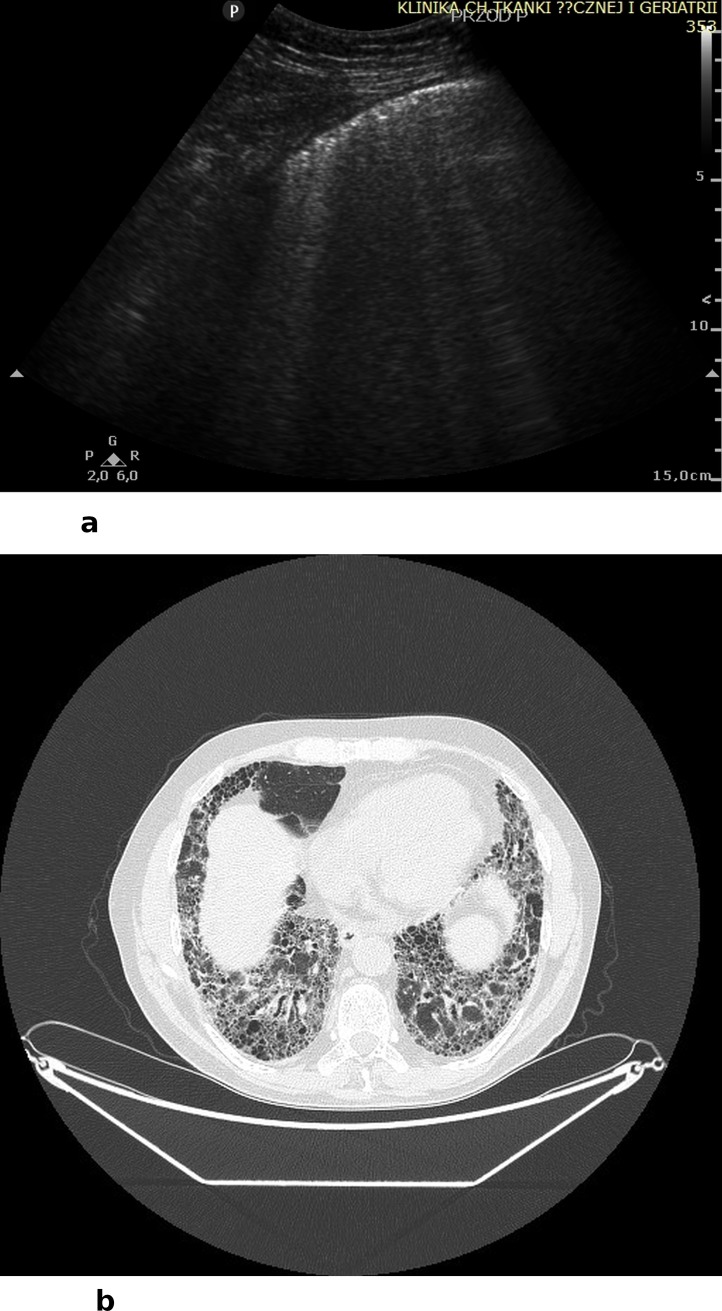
A) LUS: Irregular pleural line, fragmentarily visualized, with B-line artifacts; ILD secondary to MPO-ANCA-positive vasculitis, B) CT: Bilateral honeycombing, features of pulmonary fibrosis secondary to MPO-ANCA-positive vasculitis.
